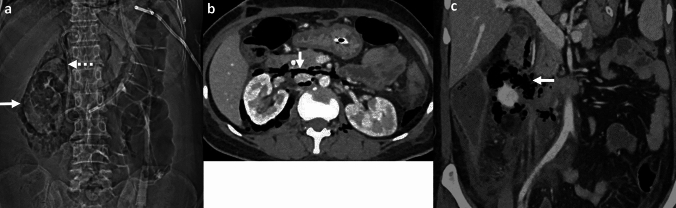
Pneumoretroperitoneum as an imaging pitfall. A 47-year-old with post-ERCP abdominal pain. CT topogram image (a) shows veiled kidney sign (solid white arrow, a) and ERCP stent (dotted white arrow, a). CT images show air in the right retroperitoneum crossing midline (solid white arrows, b, c). The pneumoretroperitoneum was secondary to duodenal perforation
